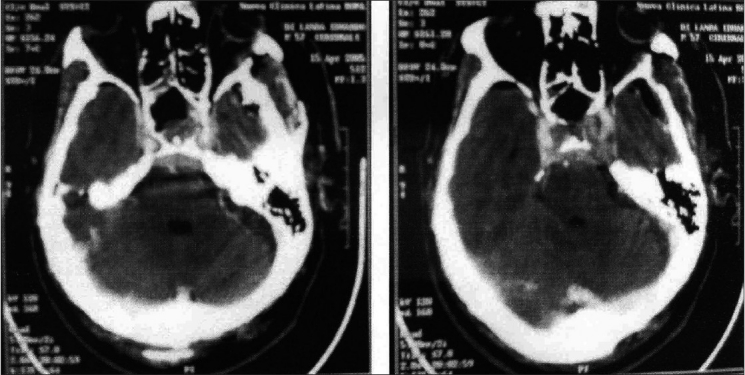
Same case as Figure 1. Immediate postoperative CT scan, before Figure 3a and after Figure 3b, contrast enhancement shows apparent total tumor removal
